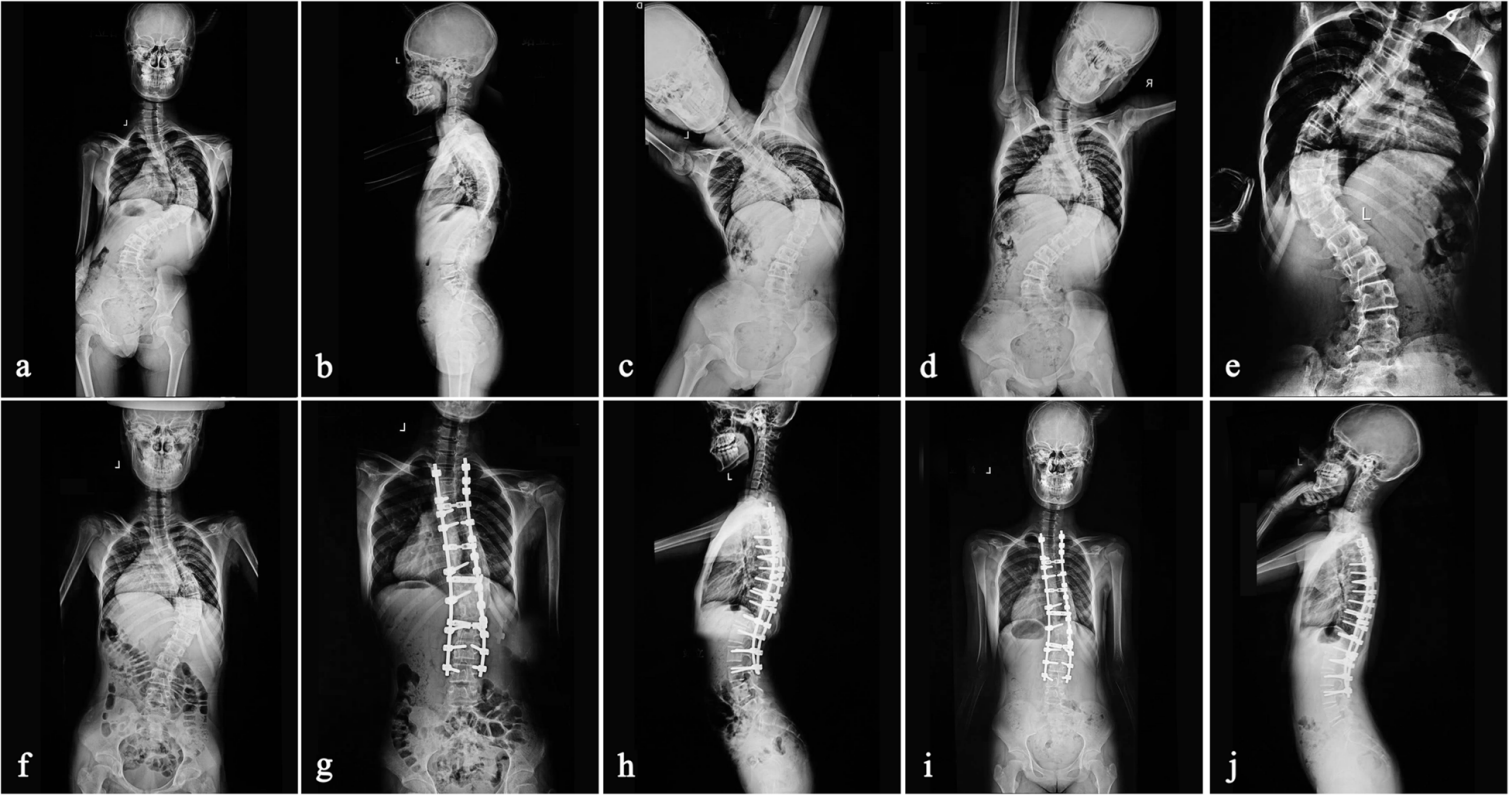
A 15-year-old female patient with Marfan syndrome had a scoliosis of 92° (a) and a kyphosis of 76° (b). Major coronal curve decreased to 80° (d) at side bending radiograph and then decreased to 72° (e) at fulcrum radiograph. After a 3-week traction, scoliosis was corrected to 58° (f). Postoperative standing radiograph demonstrated scoliosis was corrected to 24° (g), and kyphosis to 18° (h), and the 2-year follow-up shows the scoliosis was 26° (i), and kyphosis to 20° (j)
